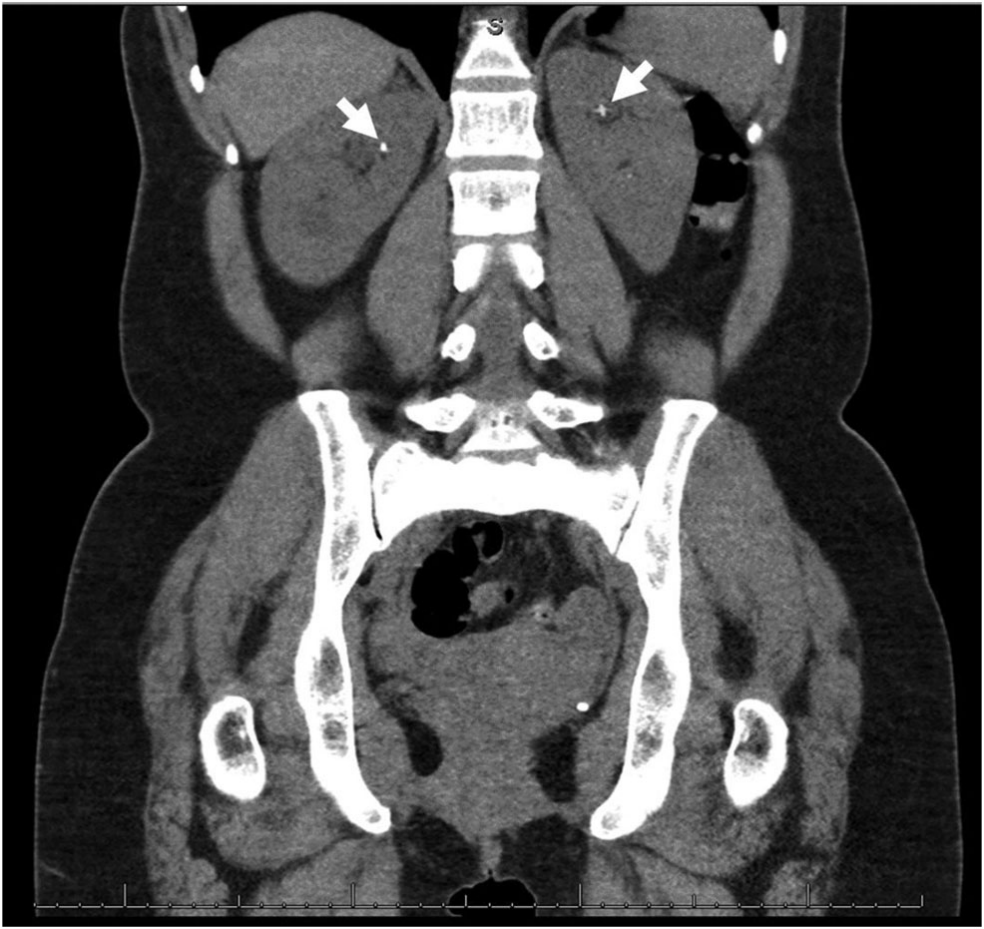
CT of kidneys, ureters and bladder (coronal view) showing bilateral renal calculi (case 2)
